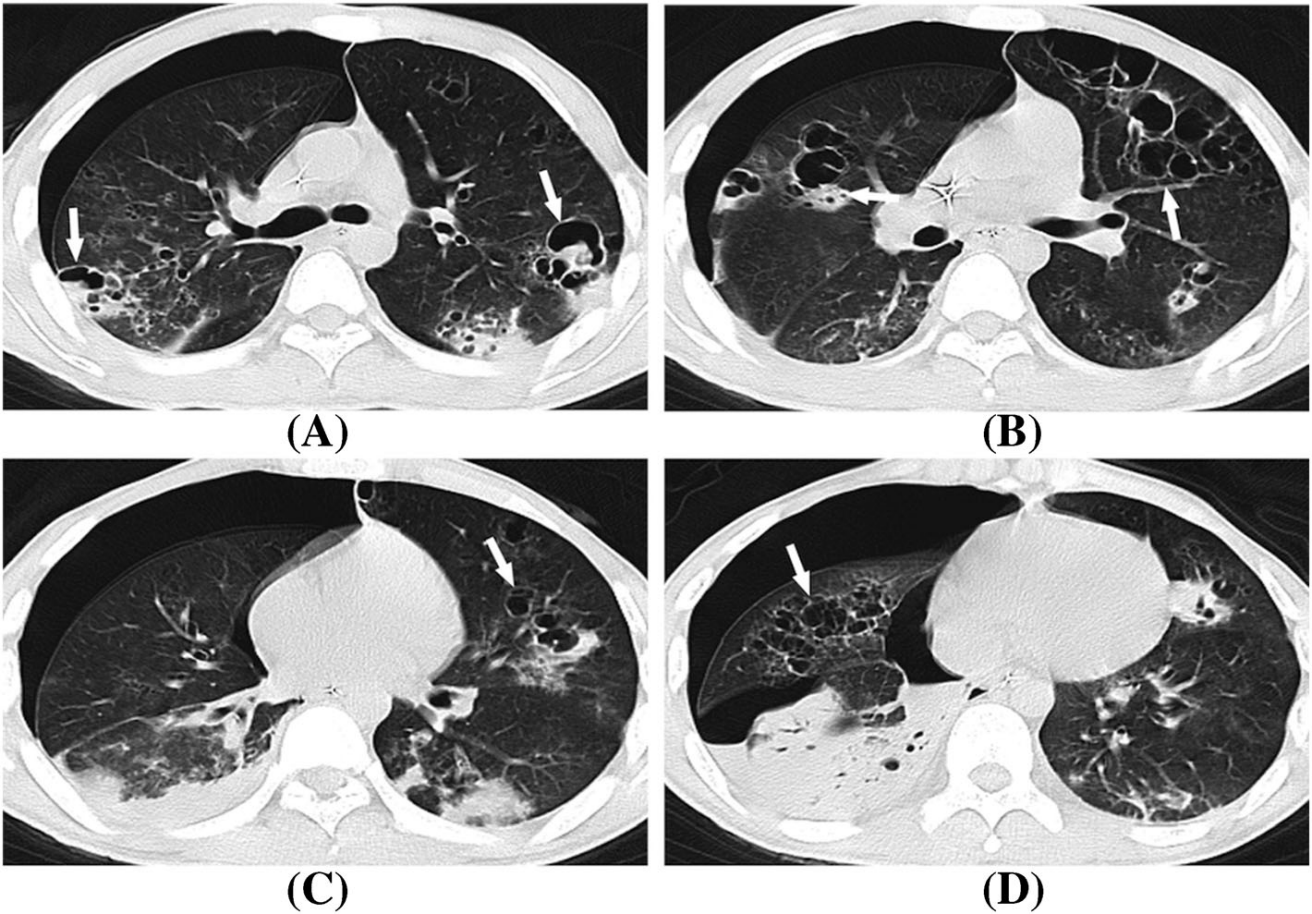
A 19‐year‐old man with critical H1N1 pneumonia, presented with fever, cough, and disturbance of consciousness. Axial CT showed multiple pneumatoceles (white arrows) in bilateral lungs and right hydropneumothorax within 17 days after the onset.
